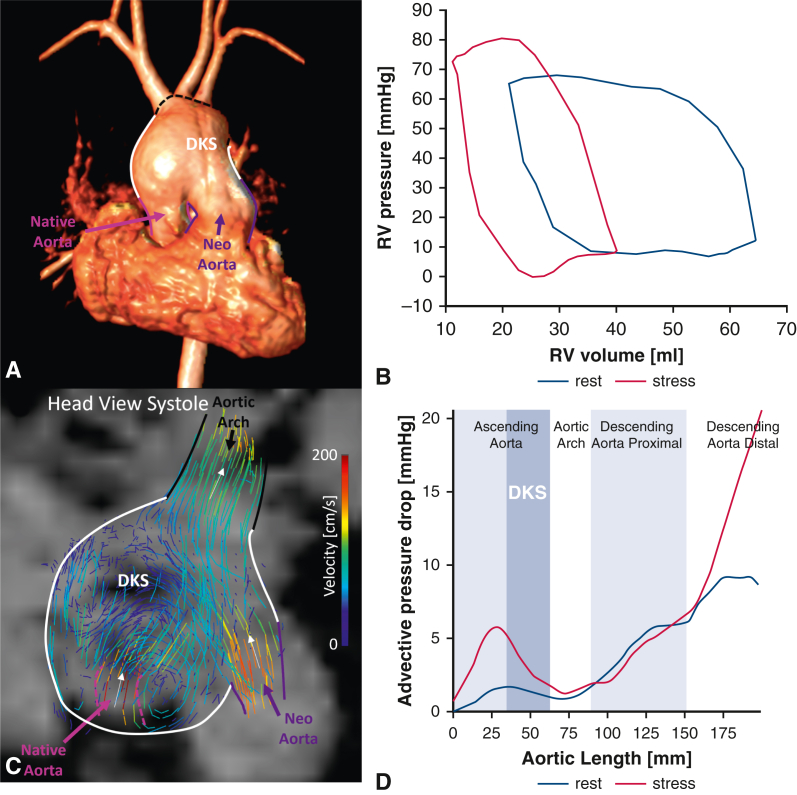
A, Three-dimensional MRI angiography of the DKS anastomosis. B, Pressure–volume loops of the systemic right ventricle at rest and under pharmacologic stress. C, Four-dimensional flow MRI streamlines showing the vortex in DKS region at peak systole. D, Comparison of conduit function at rest and stress, assessed by the advective pressure drop along the aortic length at peak systole.^3^*MRI*, Magnetic resonance imaging; *DKS*, Damus–Kaye–Stansel.
